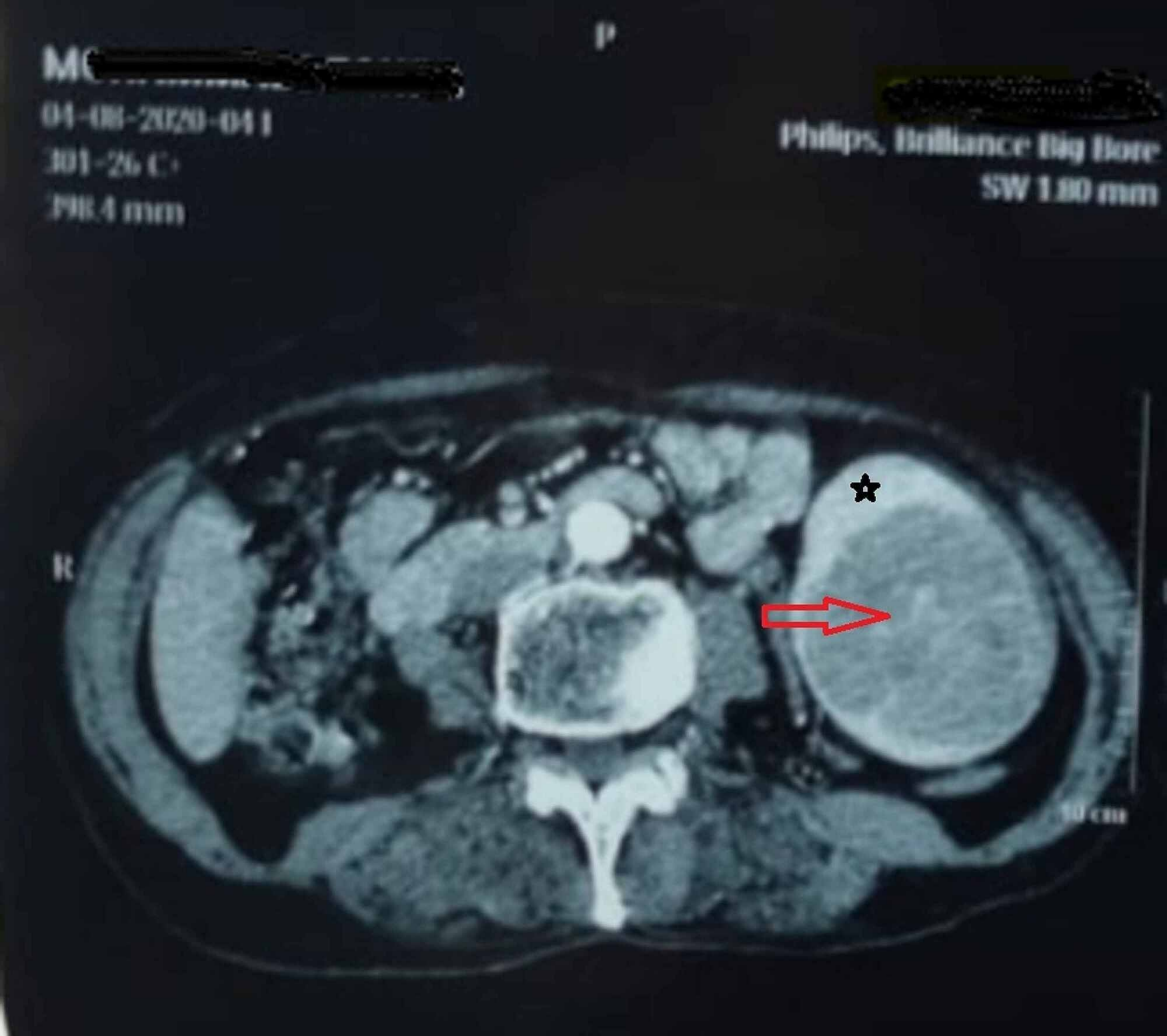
CT scan imaging revealing an inferior splenic hemangioma. Red arrow: Hemangioma Black star: Spleen
